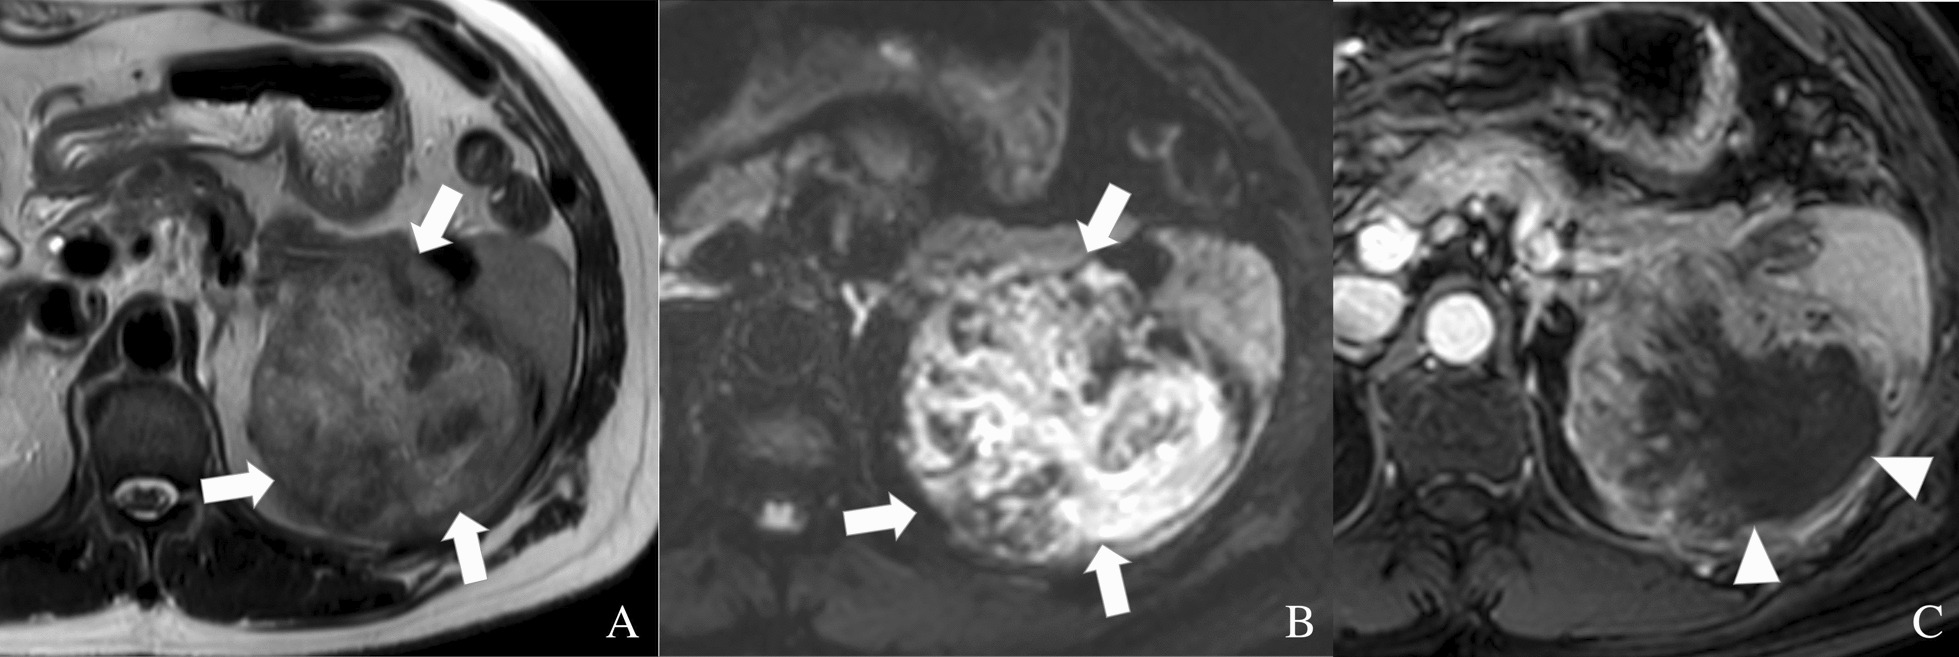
Abdominal MRI showing a large mass with heterogenous intermediate T2-signal (arrows) (A), heterogenous restricted diffusion (arrows) (B), and heterogenous peripheral enhancement on T1 fat sat post-contrast (arrowheads) imaging (C) involving the pancreatic tail and spleen. Overall findings demonstrate a relatively circumscribed, large mass with solid and fluid components and peripheral solid enhancement suggestive of high cellularity and central necrosis invading the splenic parenchyma
